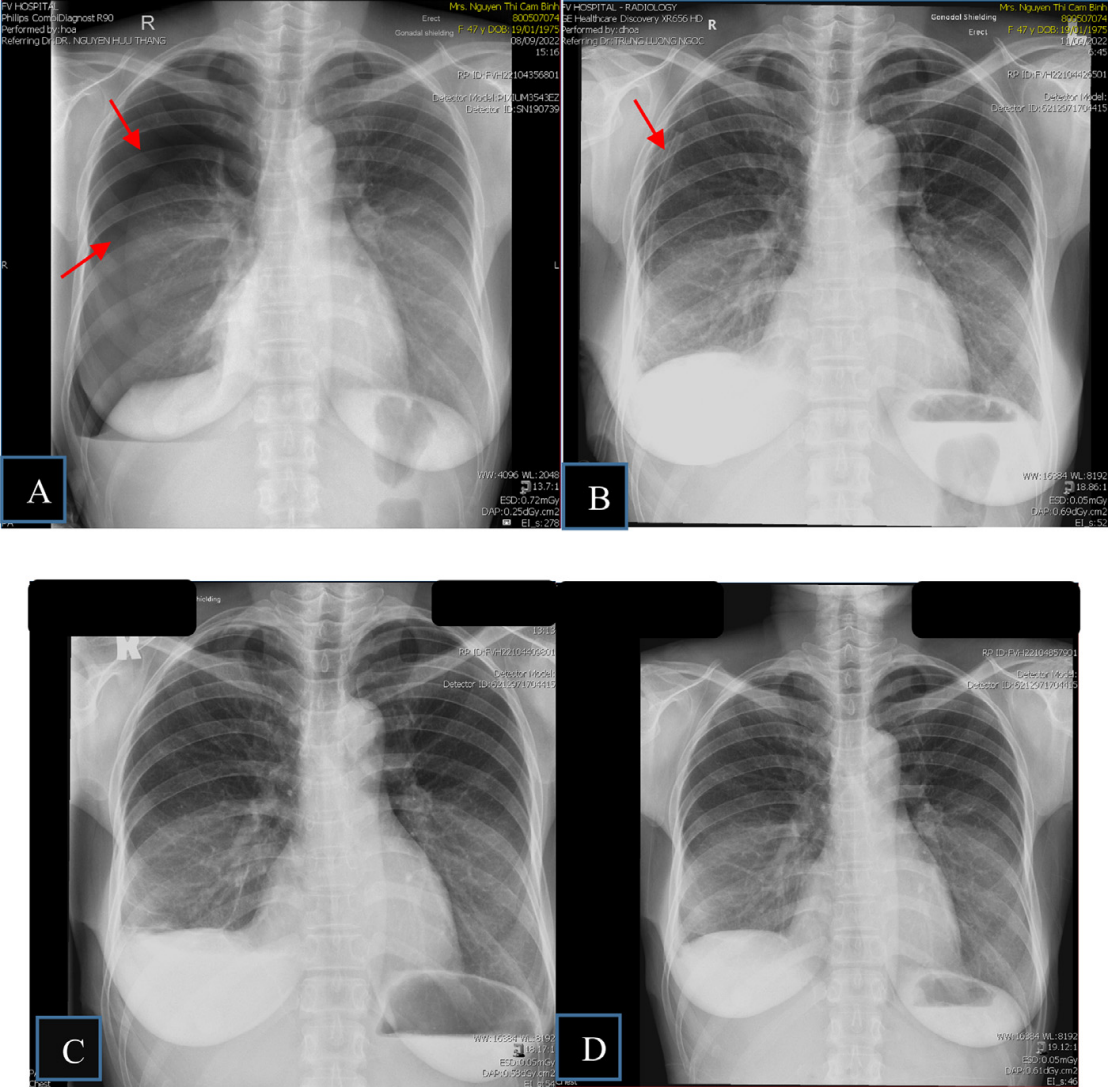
Chest X-ray results. (A) X-ray on September 8, 2022: Significant right-sided pneumothorax. (B) X-ray on September 11, 2022: Right-sided pleural drainage tube. (C) X-ray on September 20, 2022: No pneumothorax detected. (D) X-ray on September 29, 2022: No pneumothorax detected.
